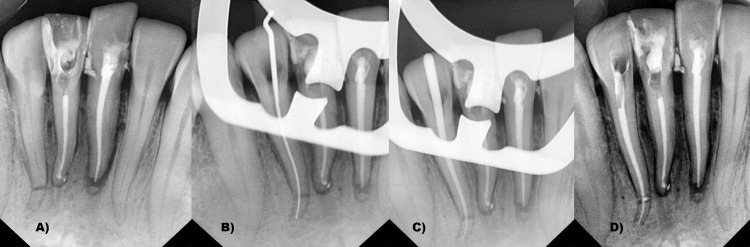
Endodontic therapy and unifying apical and coronal segments in apically fractured 42 A) Preoperative radiograph; B) working length estimation; C) master cone; D) unifying apical and coronal segments
